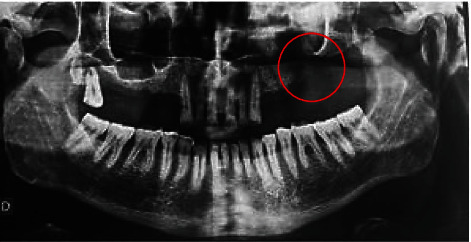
Panoramic radiography. The maxillary defect is shown with the circle above.
